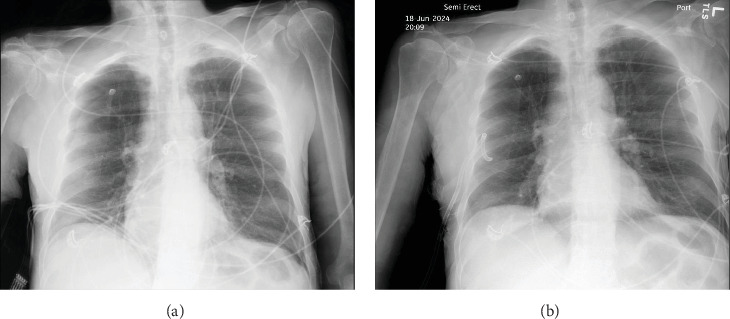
(a) Chest X-ray on arrival demonstrating a right-sided pneumothorax. (b) Chest X-ray demonstrating reexpansion of lung after placement of thoracostomy.
